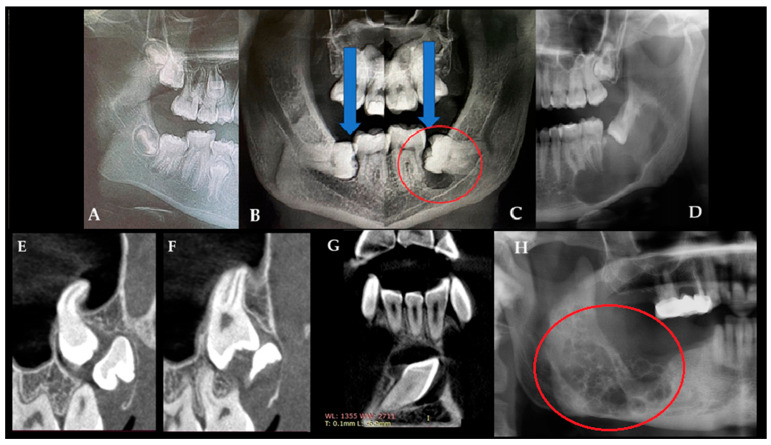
Many radiolucent lesions with well-defined borders represent cysts within the oral cavity. Each developing tooth is surrounded by a small, round radiolucent area known as the dental follicle, which is typically well defined and less than 4 mm in diameter (A). During growth, maturation, and tooth eruption, the dental follicle can remain, reduce its shape, disappear in time (blue arrows indicate the dental follicle on the left side that progressively regressed and disappeared), or form a follicular cyst (red circle). This situation occurs mostly when it is associated with totally or partially unerupted teeth (B,C). Panoramic radiography (OPG) is a useful initial screening tool and can identify various cystic and cyst-like jaw lesions of different sizes, shapes, and locations ((D)—unicystic ameloblastoma, UA). In general, retained teeth and associated cystic cavities should be further evaluated using CBCT (E–G). For comparison, a representative OPG of an odontogenic myxoma is shown in (H), demonstrating typical multilocular, lobulated (the red circle highlights the soap-bubble appearance) radiolucency with internal septations, a classic pattern that helps differentiate myxoma from simple cystic lesions. On the other hand, not all found cyst-like appearances on a routine OPG are a cyst; in these cases, CBCT is recommended to ensure that the dental follicle and formation of follicular cysts or any other cyst-like lesion do not have any worrisome symptoms, such as the following: bone erosion, teeth resorption, cortical swelling, any presence of bone irregularities, septa, or a mixed radiolucent–radiopaque appearance that might suggest the formation of a possible odontogenic tumor (abbreviation from image (G): I—inferior (lower border of mandible); WL/T (mm)—location of the follicle in CBCT in millimeters).
